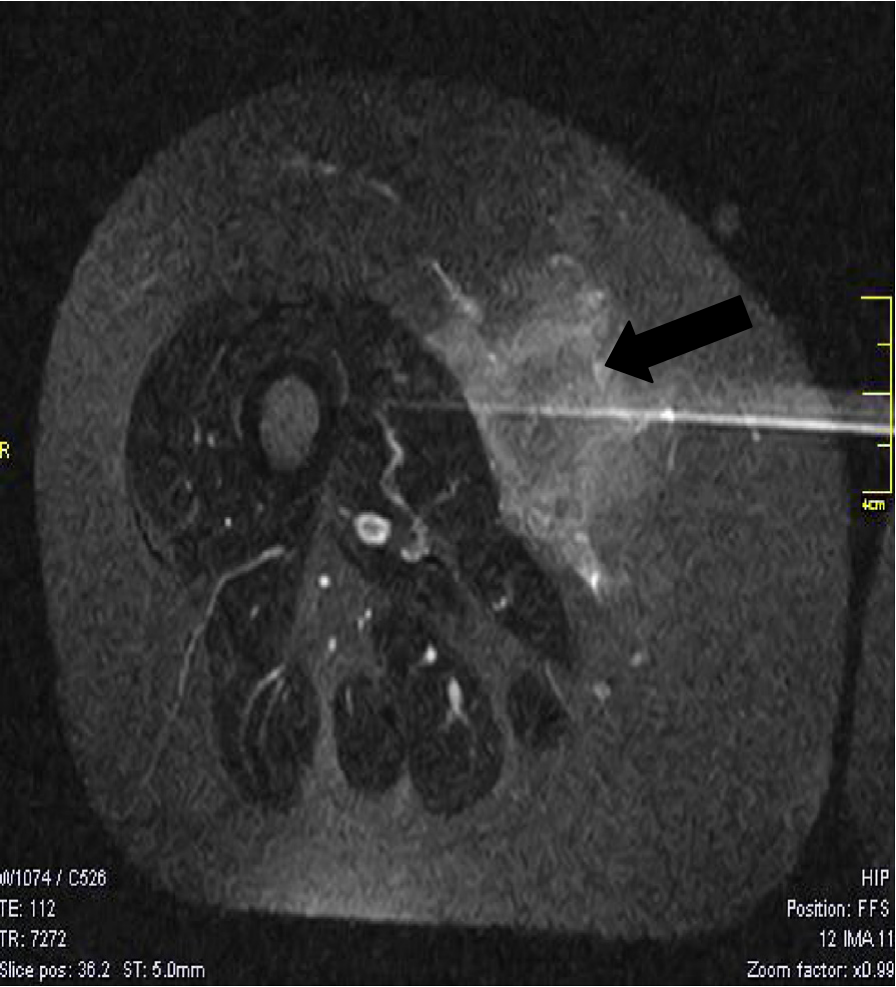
Large radiolucent mass in the right medial thigh. T1-weighted transverse magnetic resonance image of the right lower extremity demonstrating a large radiolucent mass in the right medial thigh (black arrow).
